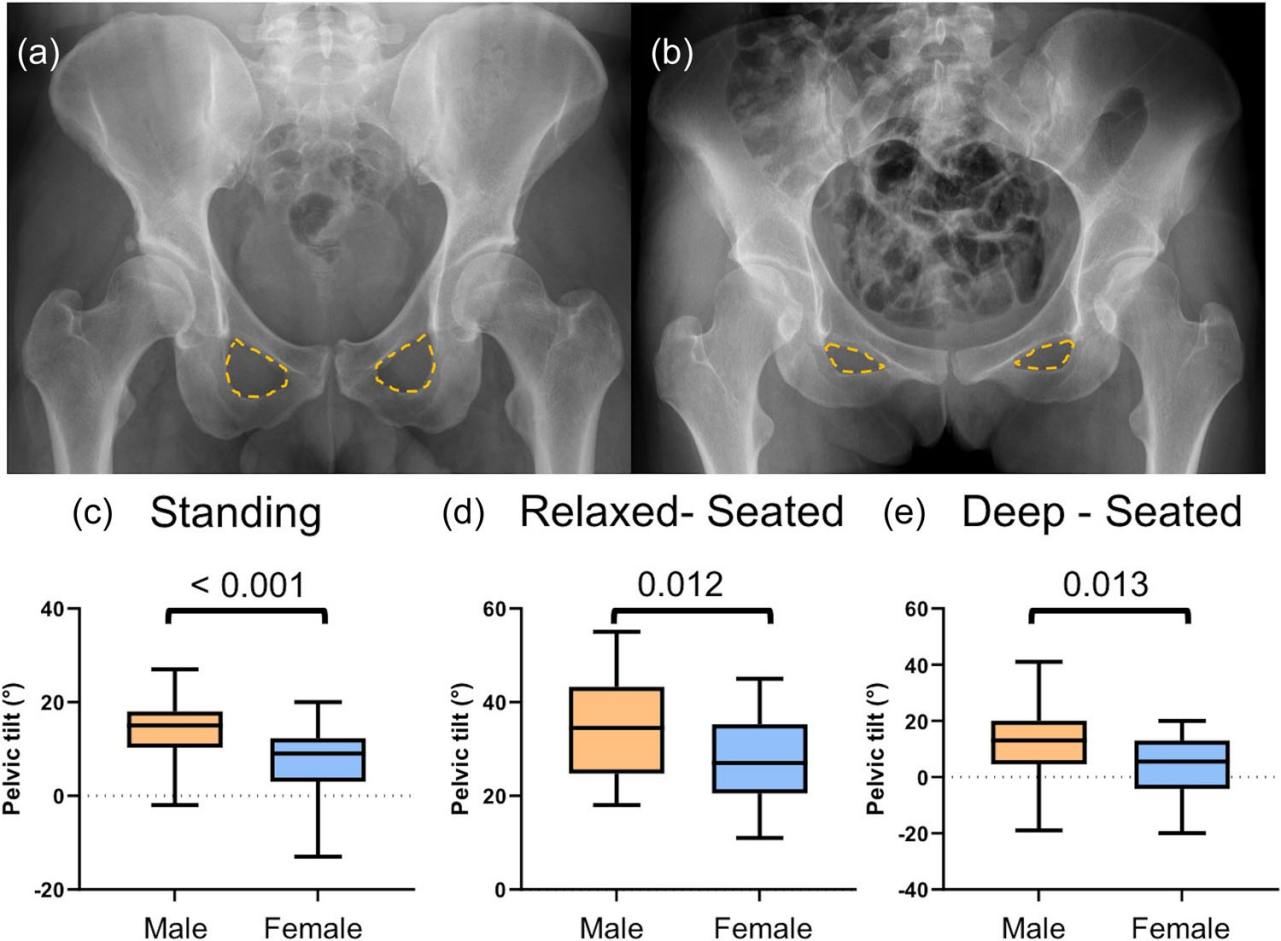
Sex‐related difference in PT. (a and b) The obturator foramen projection (yellow line) is the first indicator for differences in PT between males (a) and females (b) on anteroposterior pelvic radiographs. (c–e) Sagittal PT in standing, relaxed‐seated and deep‐seated positions. Mann–Whitney U‐test, *p* < 0.05.
